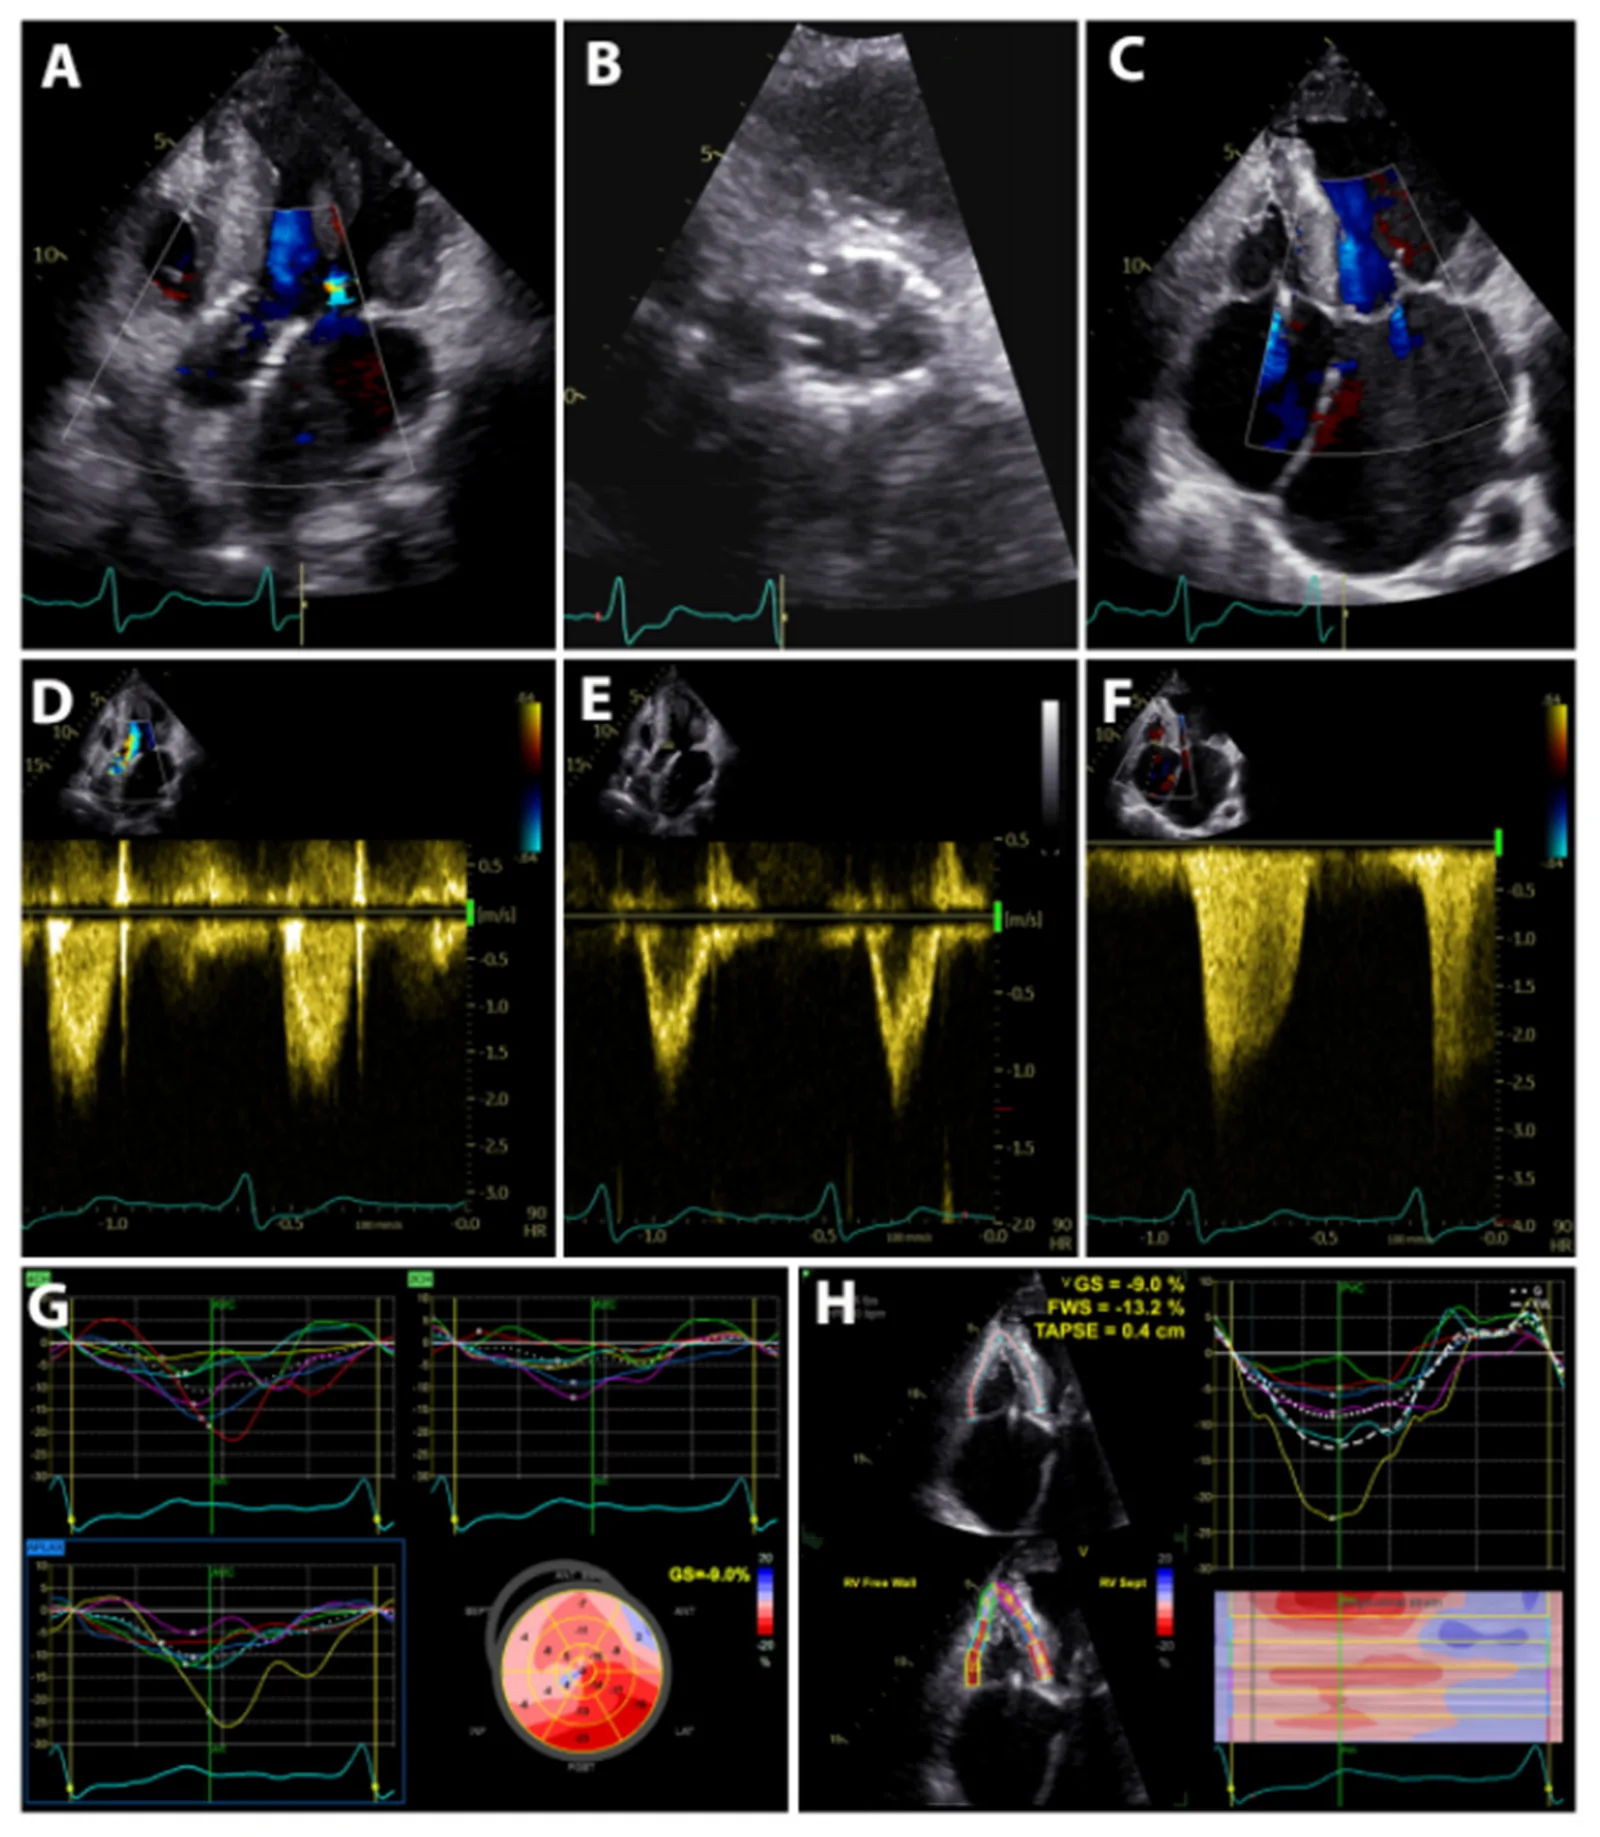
Three-month TTE follow-up after TAVI. (A) Apical 5 chambers view color Doppler view shows a normally functioning Edwards SAPIEN 3 prosthesis without significant residual aortic regurgitation. (B) Parasternal short-axis view of the prosthetic valve. (C) Apical four-chamber color Doppler view demonstrating mild residual mitral and tricuspid regurgitation. (D) Pulsed-wave Doppler interrogation of the left ventricular outflow tract demonstrating normal flow velocities. (E) Pulsed-wave Doppler at the mid-ventricular septum demonstrating the absence of dynamic left ventricular outflow tract obstruction following septal myectomy and TAVI. (F) Continuous-wave Doppler interrogation of the tricuspid regurgitation jet for estimation of pulmonary artery systolic pressure (PASP). (G) Left ventricular speckle-tracking analysis demonstrating persistent impairment of global longitudinal strain. (H) Right ventricular free-wall strain analysis demonstrates persistent improvement of RV systolic dysfunction.
